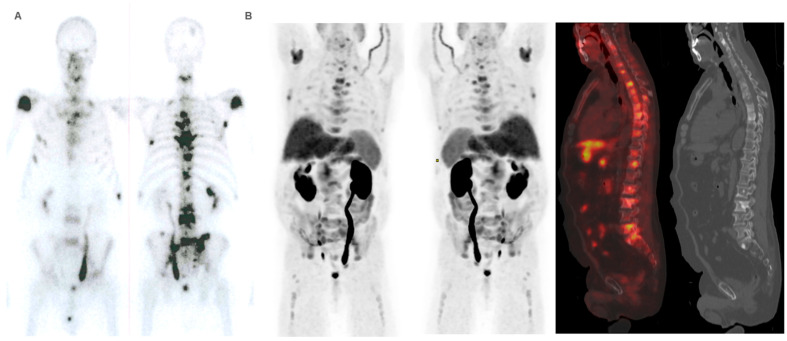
Patient ≠ 1. A 71-year-old man, diagnosed with prostate adenocarcinoma Gleason Score 9 (4 + 5), underwent prostatectomy followed by adjuvant radiotherapy to the surgical bed due to early PSA progression. Three years after diagnosis, bone metastases were detected, prompting the start of systemic treatment lines (Enzalutamide and Docetaxel). ^223^Ra was administered as the third line. At the start of treatment, the patient was in good general condition (ECOG 0), experienced pain, and had baseline PSA levels of 50.7 ng/dL, AP of 377 IU/L, and LDH of 377 IU/L. Baseline BS (A) shows polymetastatic disease (>20 lesions) affecting both the axial and extra-axial skeleton. Baseline FCH PET/CT (B) shows the presence of mixed characteristic BM, bone marrow infiltration, and uptake of the most hypermetabolic BM higher than liver and soft tissue involvement at the pelvic lymph nodes. The concordance between both studies was moderate, defining FCH PET/CT more BM with respect to BS.
